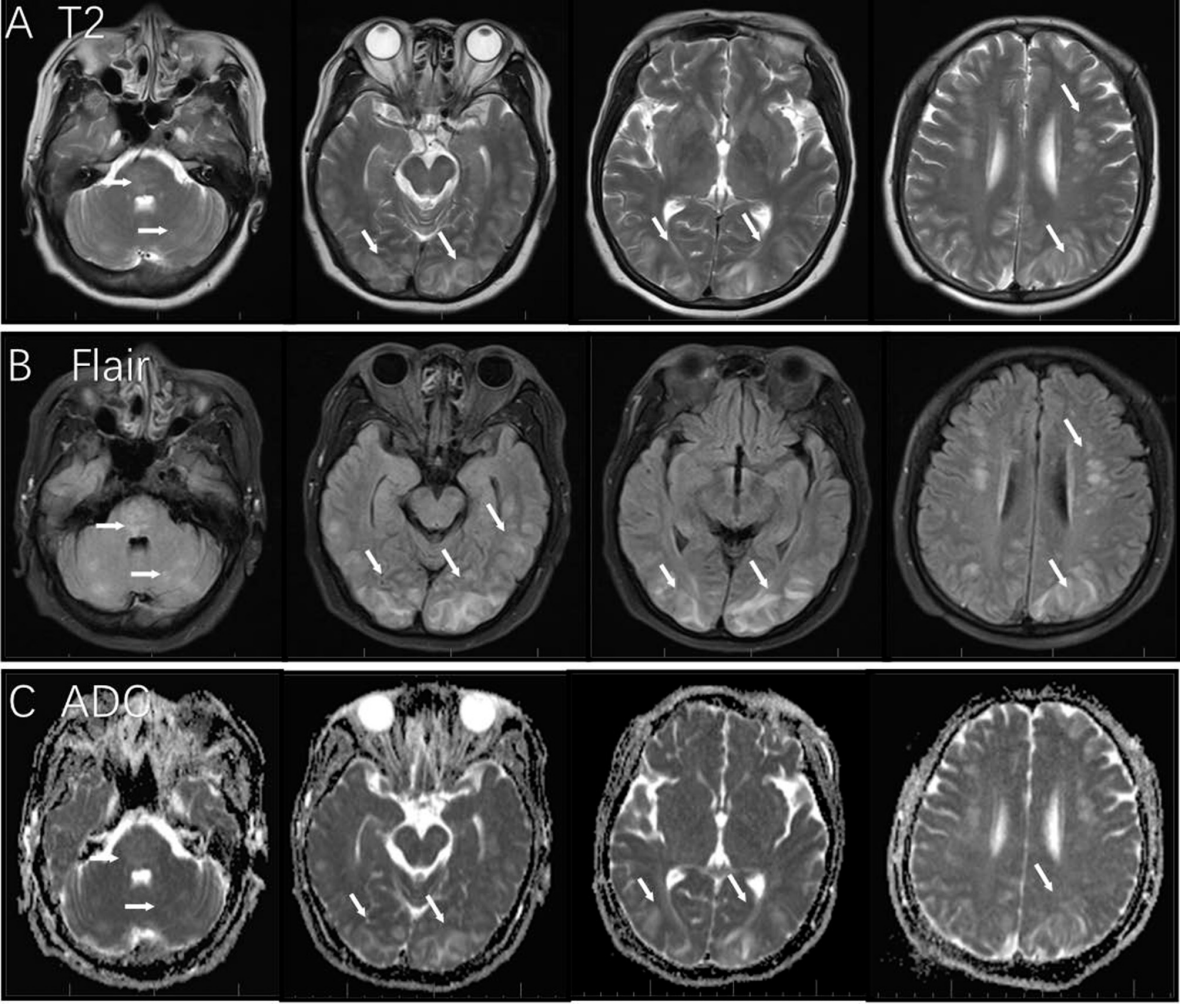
MRI findings in this case of posterior reversible encephalopathy syndrome. T2 and Flair image showed hyperintensity in the area of the cerebellum, pons, fronto-parieto-occipital areas, and corona radiate [white arrows in panels (A,B)]; bilateral parieto-occipital cortical regions showed hyperintensity in ADC [white arrows in panel (C)].
